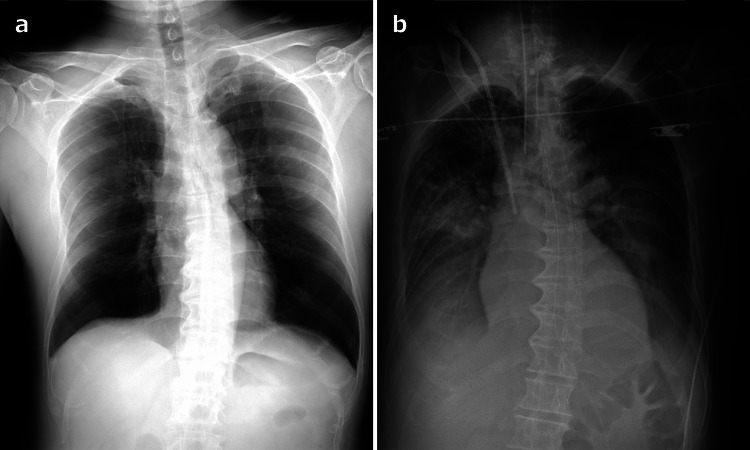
Chest radiographs obtained during hospitalization. (a) Initial chest radiograph obtained on admission was unremarkable. (b) Chest radiograph obtained on the sixth day of hospitalization showed pulmonary edema and pleural effusion.
